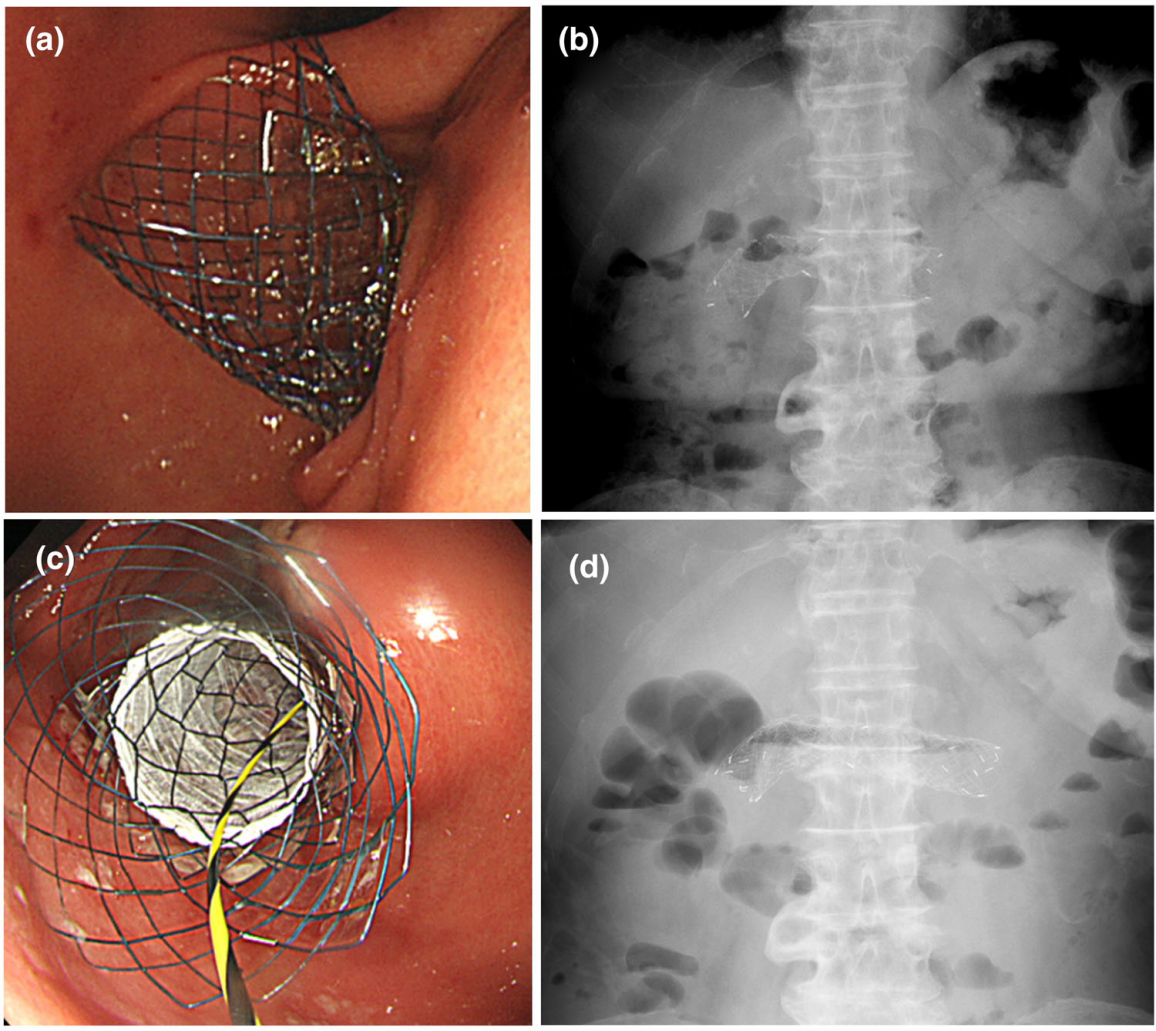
Endoscopic and radiographic images of the metallic stent; (a) the endoscopic images after metallic stent placement; (b) the radiographic image shows that the metallic stent was fully open; (c) the endoscopic images after additional metallic stent placement; (d) the radiographic image shows that the additional metallic stent was fully open
